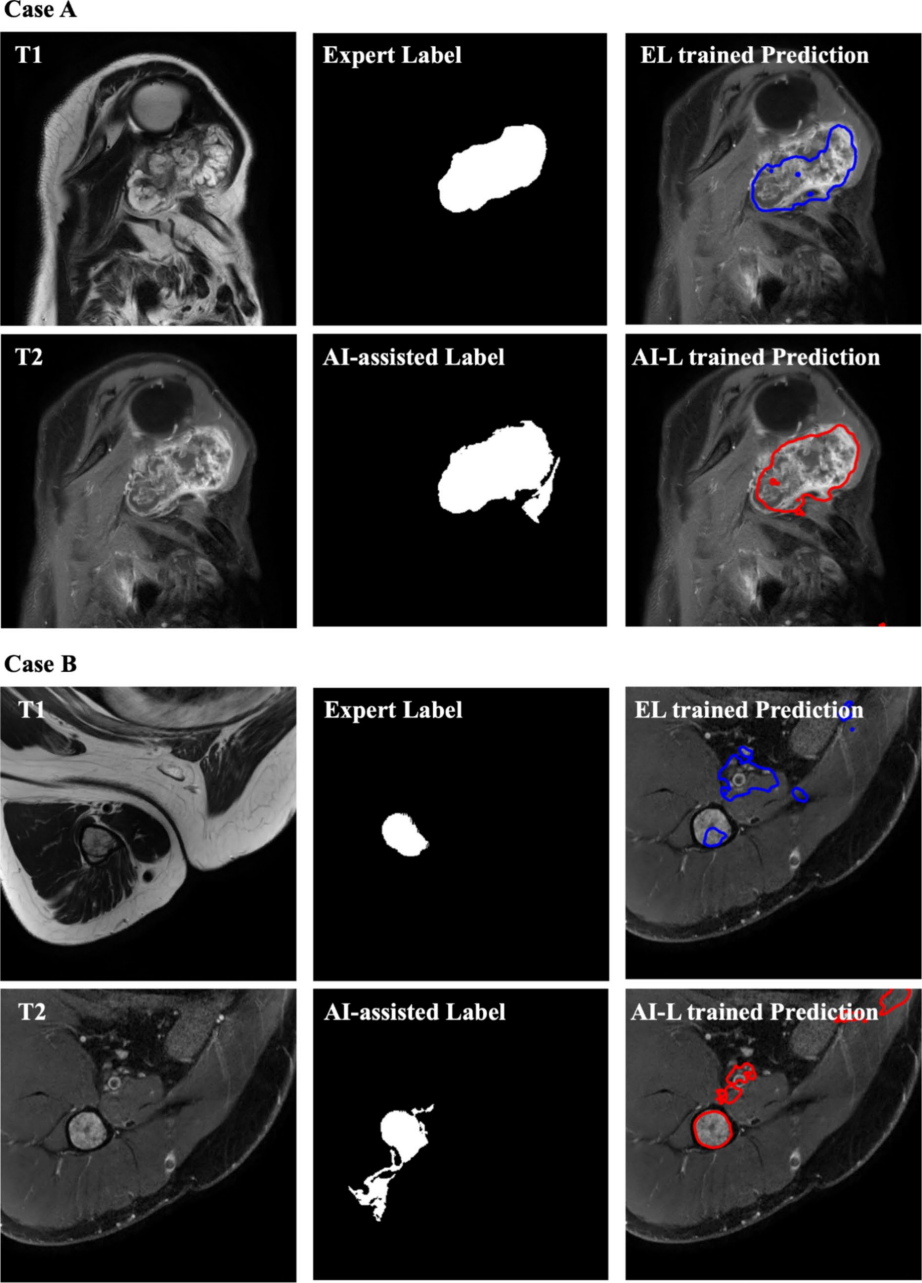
Comparison of AI-L and EL-trained model performance. This figure showcases two cases where the AI-L-trained model outperformed the EL-trained model based on a complete agreement between experts. For each case, the labels used for training are shown alongside the resulting predictions overlaid on the T2-weighted image
